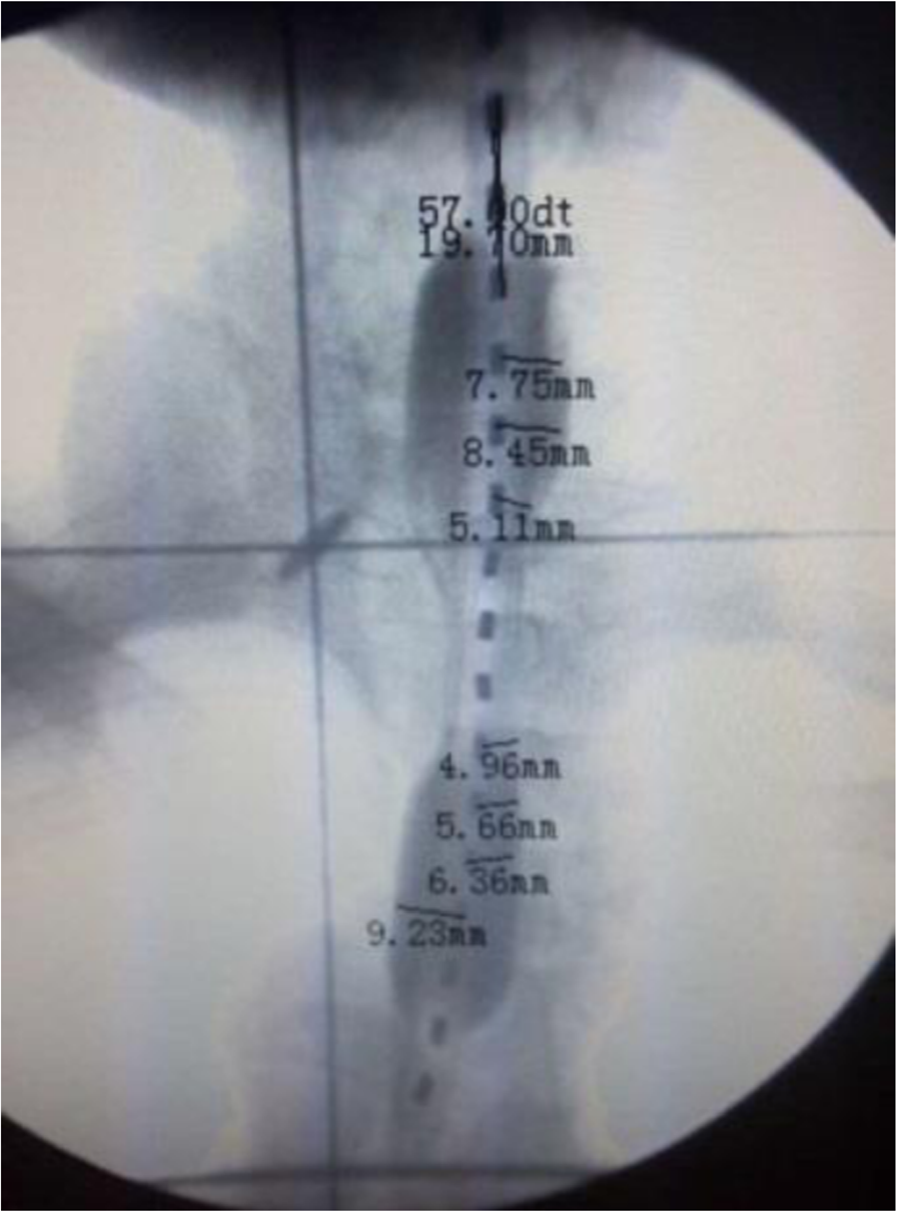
The x-ray image showing the tumor regression conditions before each of the 2–5 treatment fractions of NBT
